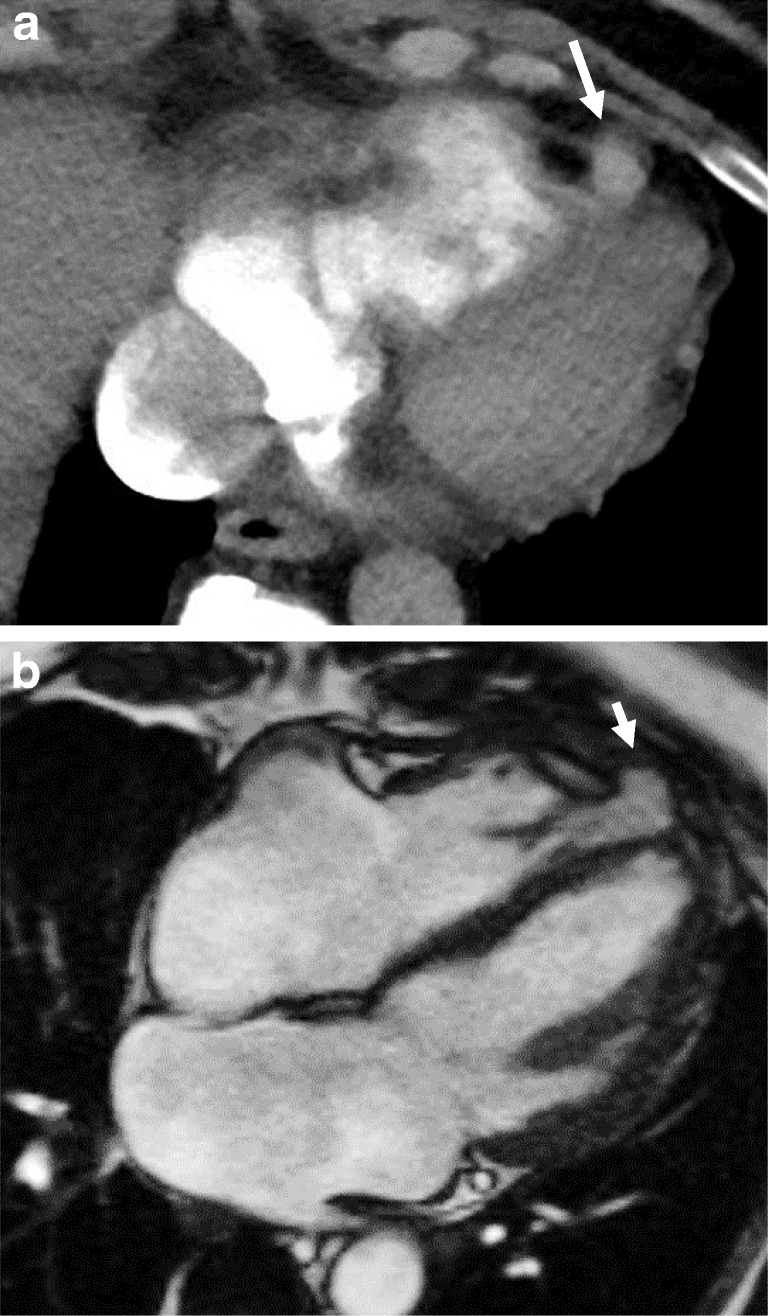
a, b CT (a) and MRI (b) images through the heart demonstrate focal hernia of the right ventricular myocardium through a congenital anterior pericardial defect (white arrow)
